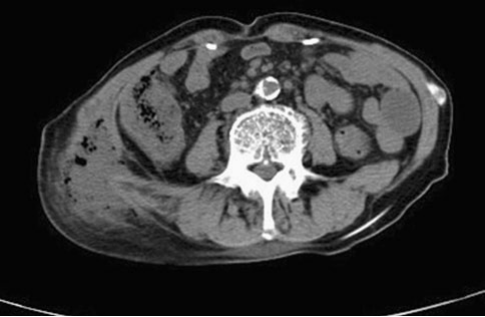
Abdominal computed tomography scan (*horizontal image*) showed gas and fluid collection from the subcutaneous layer to the retroperitoneal cavity, with inflammation extending into the adjacent soft tissue near the loin
